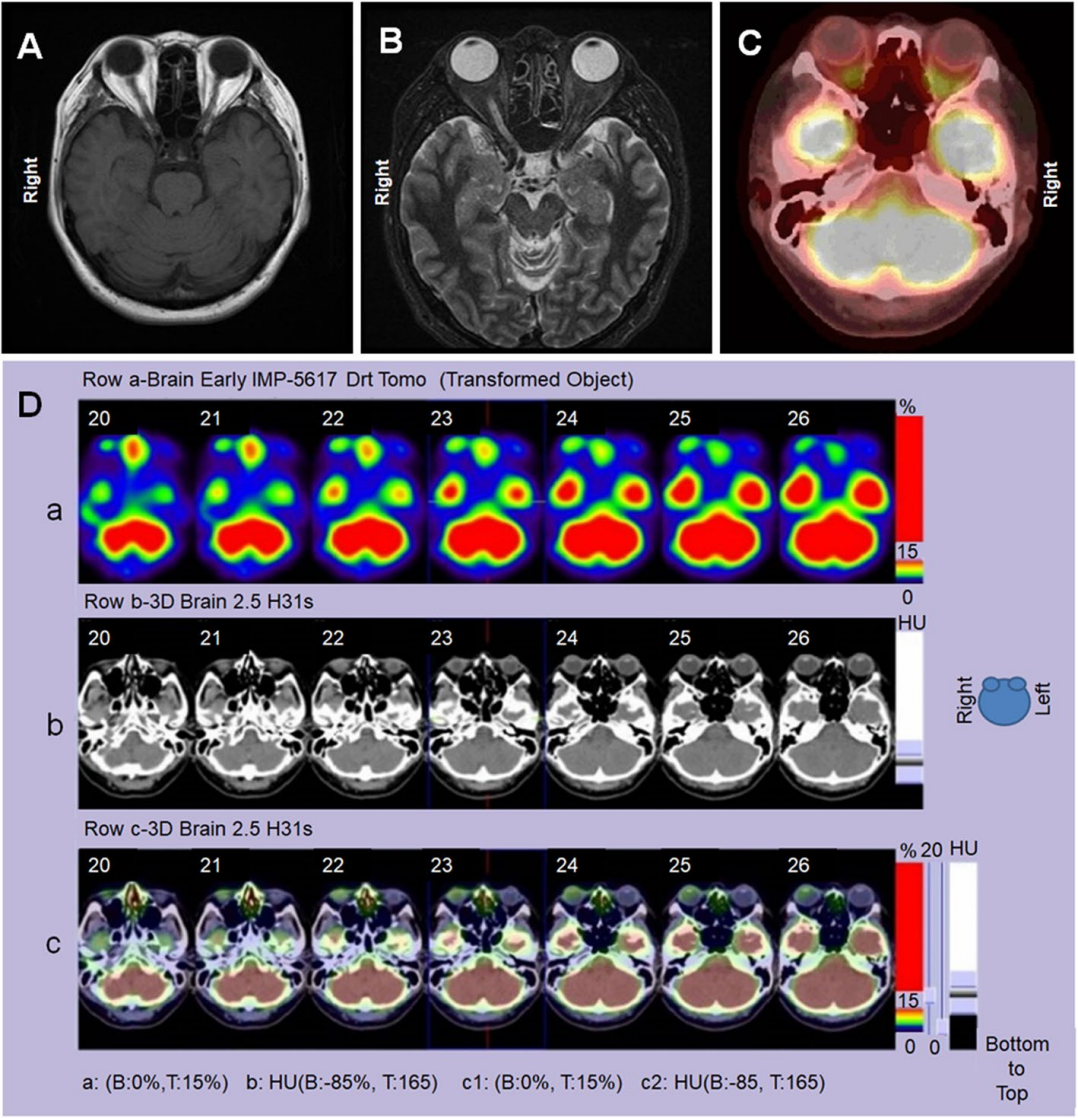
Identification of malignancy by imaging studies. A cranial MRI image shows no abnormality on a T1-weighted image (A) and a high signal in the optic nerve of the right eye on a short tau inversion recovery image, but no findings suggestive of malignancy are seen (B). A PET-CT also shows no signs of malignancy (C). Brain SPECT shows that fluorodeoxyglucose is slightly more concentrated in the right eye than in the left eye in the early images, but no difference is seen between the left and right eyes in the late image and there is no significant accumulation in the trunk (D)
